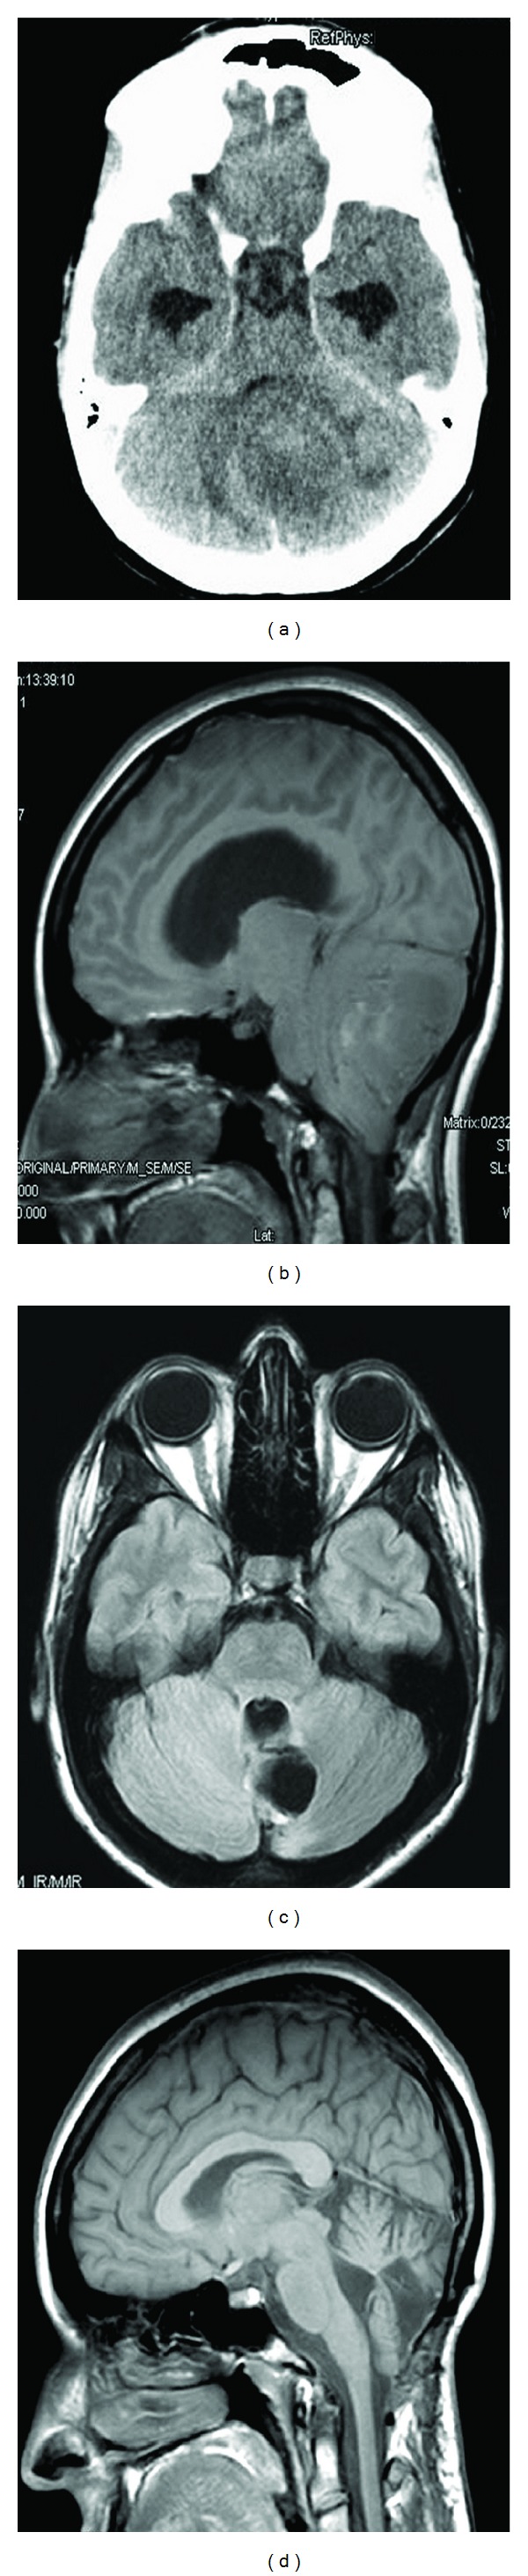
(a) CT-scan, after contrast administration, showing the cerebellar tumor, with peritumoral edema and hydrocephalus. (b) T1-weighted MRI, after gadolinium administration, showing the hypointense cerebellar tumor, with areas of hyperintensity. (c) and (d) T1-weighted MRI, after contrast administration, two years after surgery. Absence of residual tumor or tumor recidive can be seen.
